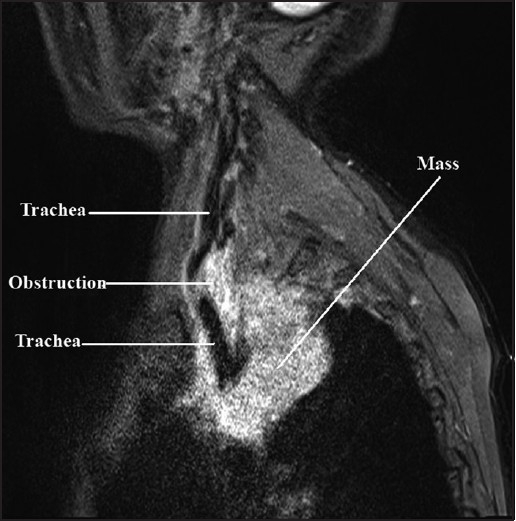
Sagittal view of MRI showing the trachea, obstruction and the mass
